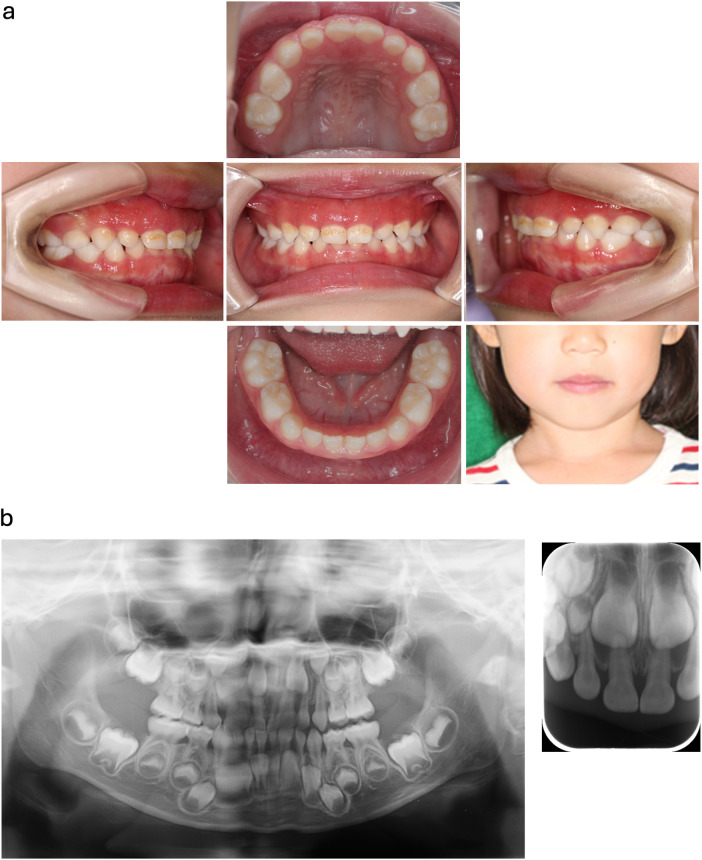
Clinical findings at the initial visit (5 years 4 months old). (a) Intraoral photograph showing severe, diffuse gingival erythema and swelling in both the maxillary and mandibular arches. Significant plaque accumulation is visible on the cervical areas of the deciduous teeth. (b) Dental and panoramic x-rays revealing no significant alveolar bone resorption, suggesting the inflammation is primarily confined to the soft tissues.
